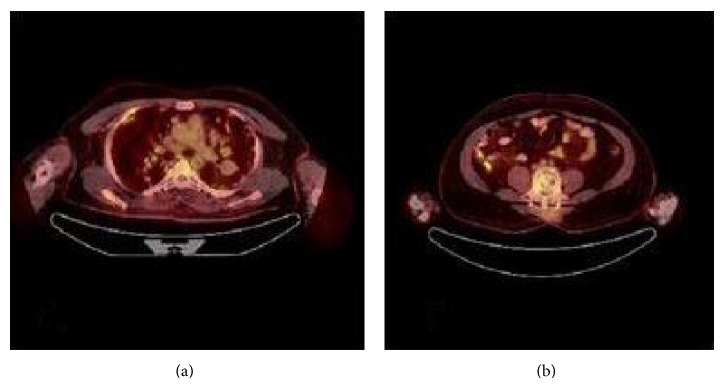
(a) Positron emission tomography with 18F-fluorodeoxyglucose (FDG) showed multiple round or oval lesions in the lung, (b) FDG uptake in midportion of L1-L2, L3 vertebral corpuses.
